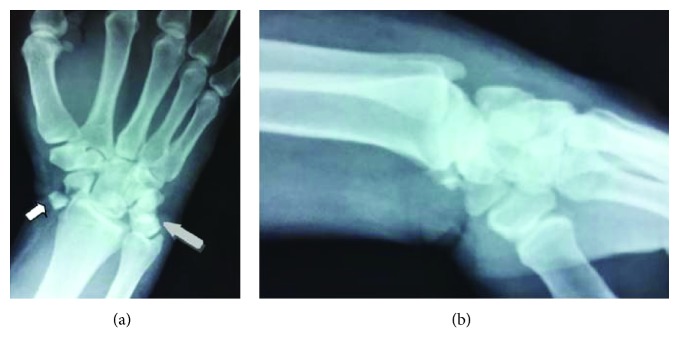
Preoperative X-rays of the left wrist (grey arrow shows the lunate, white arrow shows the scaphoid fracture).
